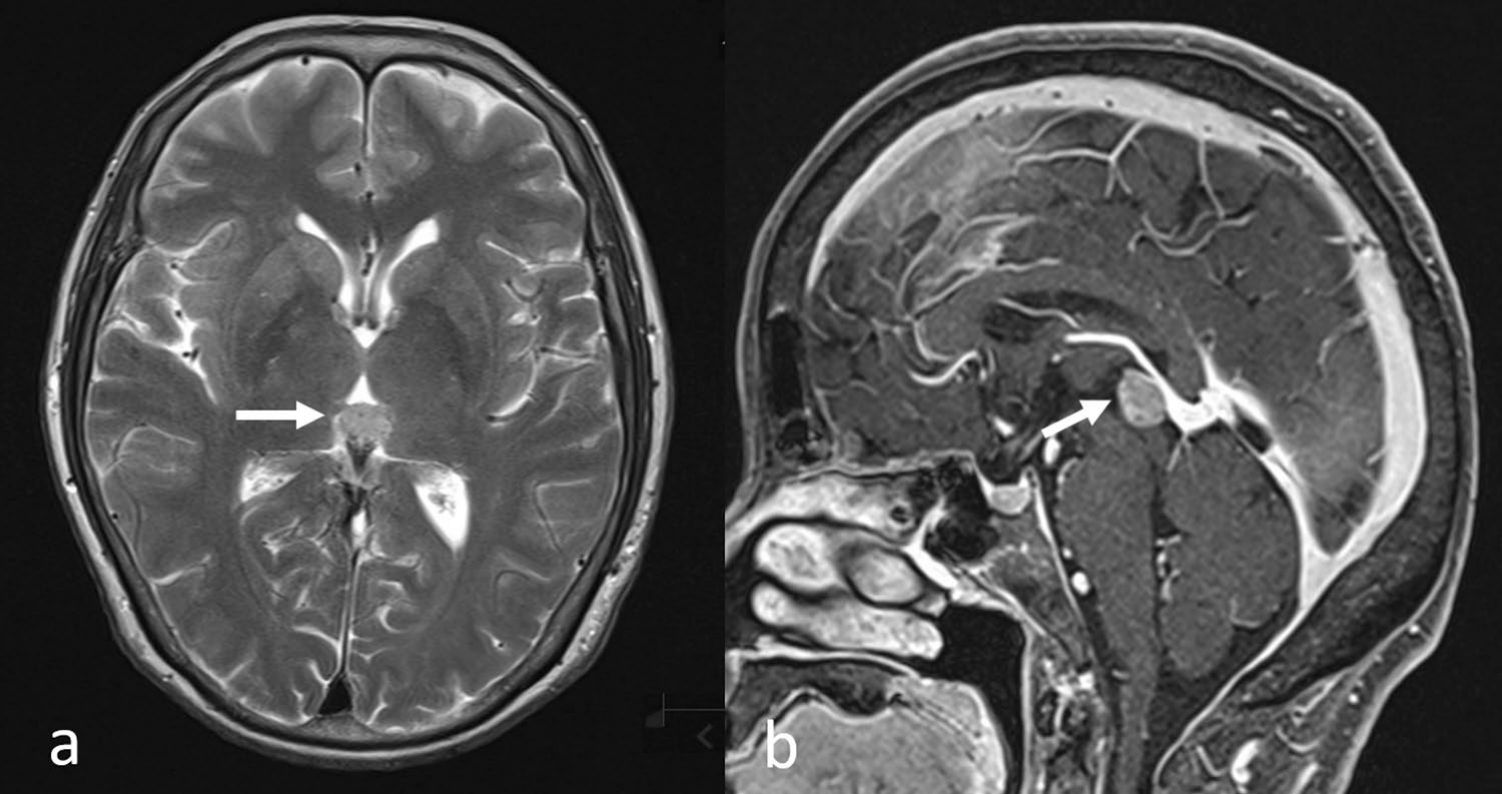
Pineal gland metastasis in a patient with lung adenocarcinoma. T2-weighted image a shows a mild hyperintense mass in the pineal area (arrow); sagittal contrast-enhanced 3D fat suppressed T1-weighted image b shows the pineal tumor with homogeneous enhancement (arrow)
